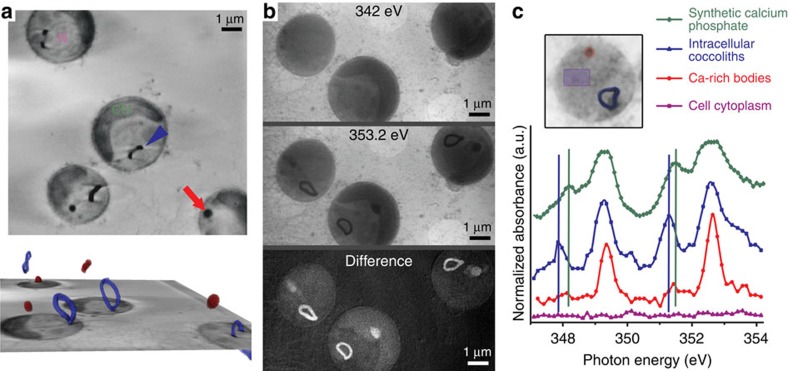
Cryo-X-ray imaging reveals concentrated calcium pools in *E. huxleyi*
